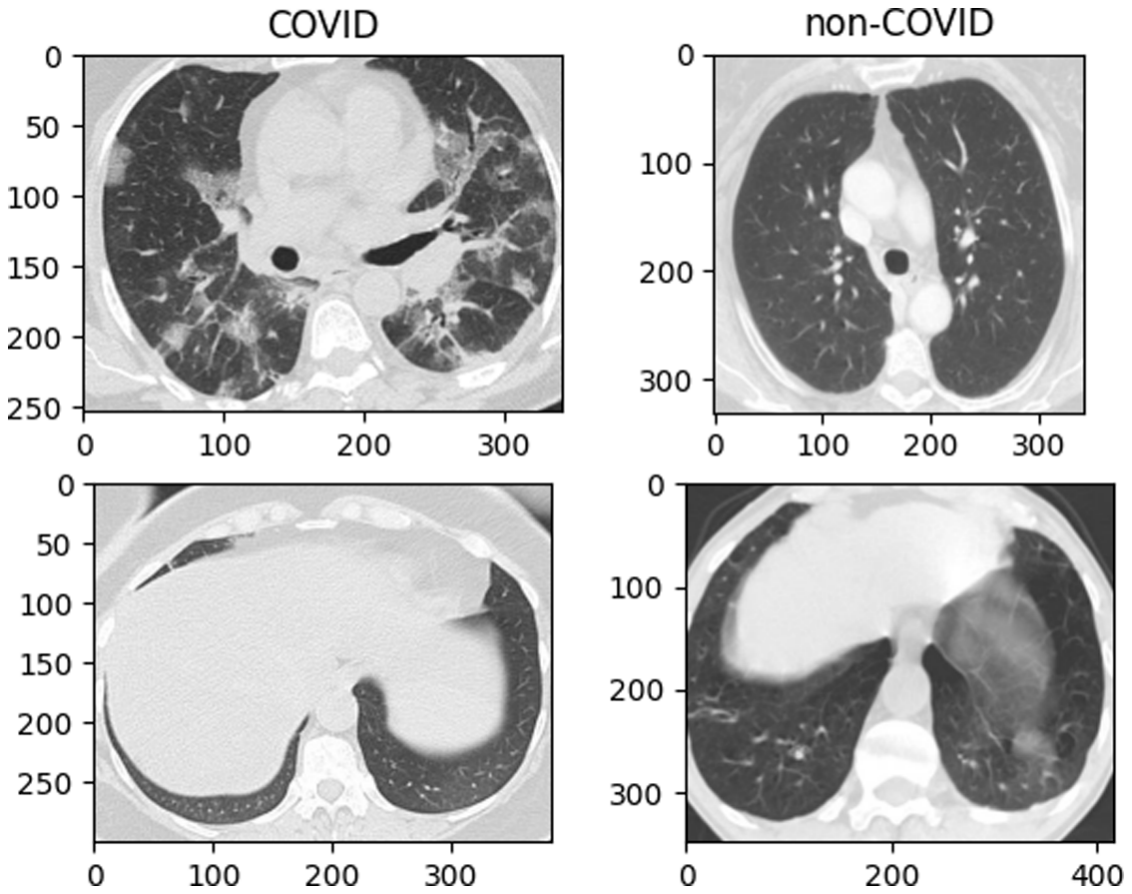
Example fundus images from the SARS-CoV-2 CT-Scan dataset.
